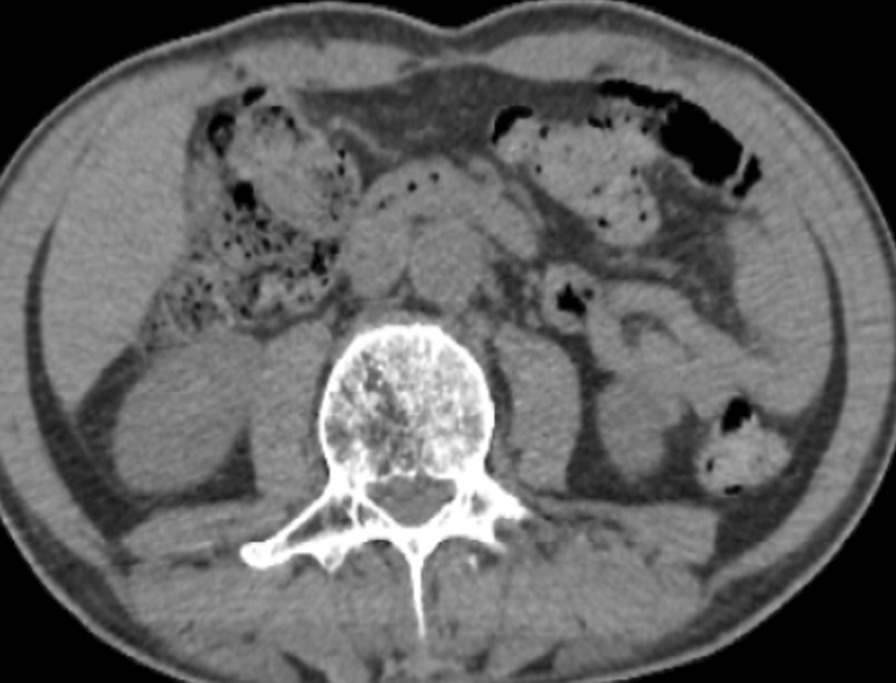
Spontaneous contrast abdominal CT scan (performed after the second bolus of cyclophosphamide) shows clear regression of periaortic tissue infiltrate
